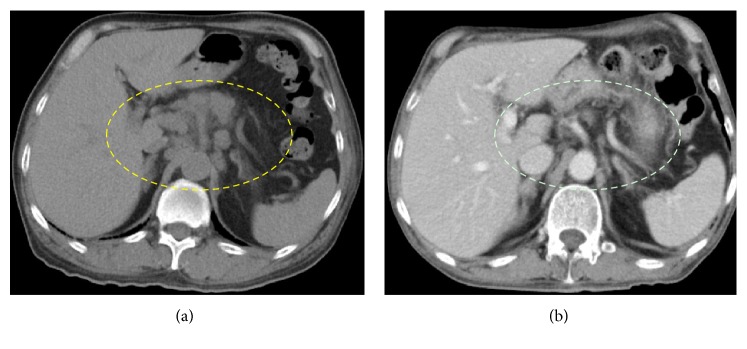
(a) Abdominal CT showing enlarged para-aortic lymph nodes. (b) After four courses of EP, enlarged lymph nodes have completely disappeared.
